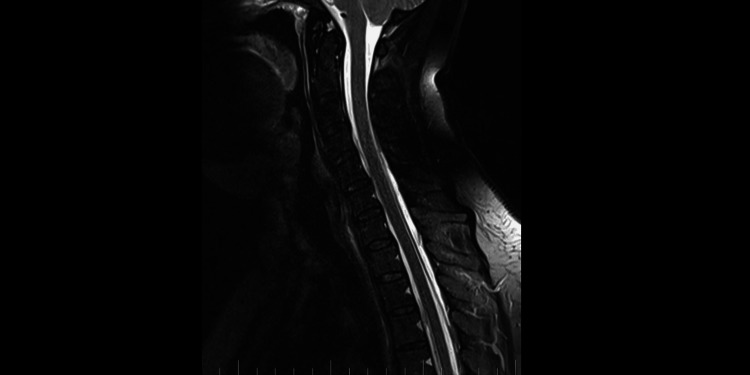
C spine MRI on September 4, 2023. Sagittal T2-weighted images showing total spinal longitudinal extradural fluid collection resorption.
